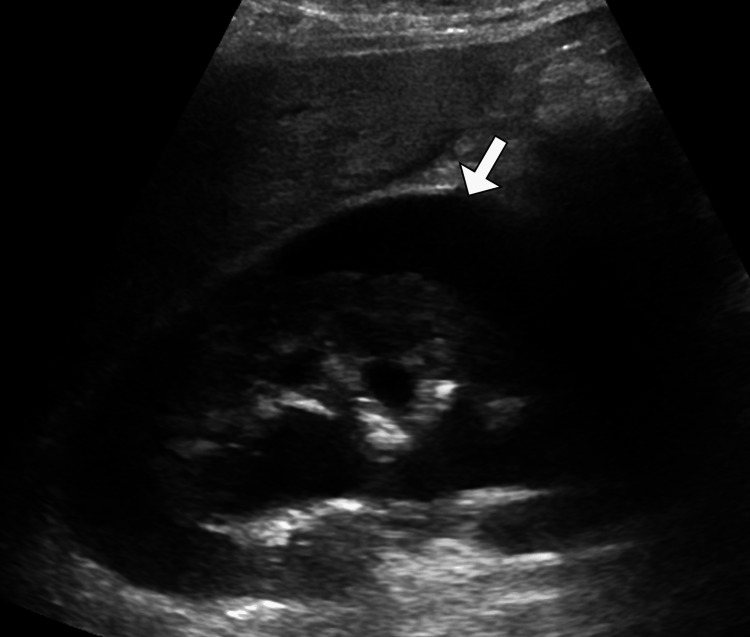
Left perirenal collection on greyscale ultrasound. Greyscale ultrasound image of the left kidney demonstrates a well-defined hypoechoic perirenal collection (arrow) surrounding the renal capsule. The appearance is consistent with a perirenal fluid collection in the clinical context of idiopathic intracranial hypertension.
